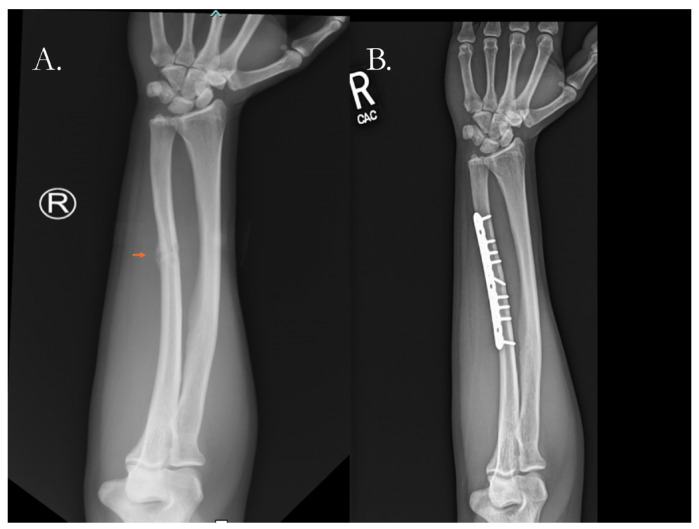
Ulnar nonunion with compression plating. 35-year-old female that presented 2 months after sustaining an ulnar fracture and was found to have nonunion of her fracture. The patient was treated with an open reduction and internal fixation with a compression plate. (A) Radiograph from when the patient was first diagnosed with nonunion (orange arrow). (B) Radiograph 3 months after fixation.
